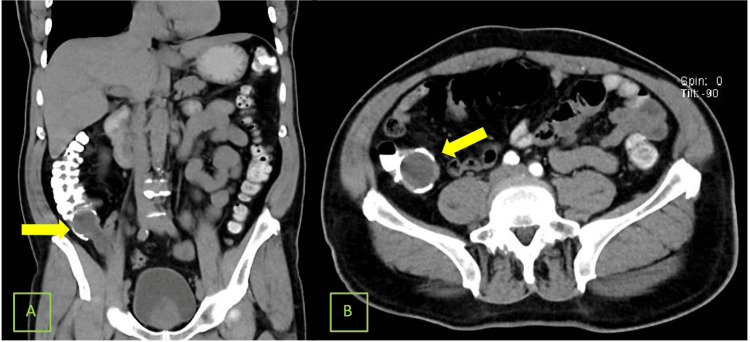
Computed tomography scan (A) Coronal section: The yellow arrow indicates at the base of the appendix an image with a soft tissue density of 25 Hounsfield units (HU). However, it is in close contact with some loops of the distal ileum, and its proximal portion is immersed in the cecum. This lesion has regular, well-defined borders. (B) Axial section: A neoplasm with dimensions of 11.4 x 3.7 x 3.45 cm does not show enhancement after the administration of the contrast medium without demonstrating streaking of adjacent fat or loss of the fat plane.
